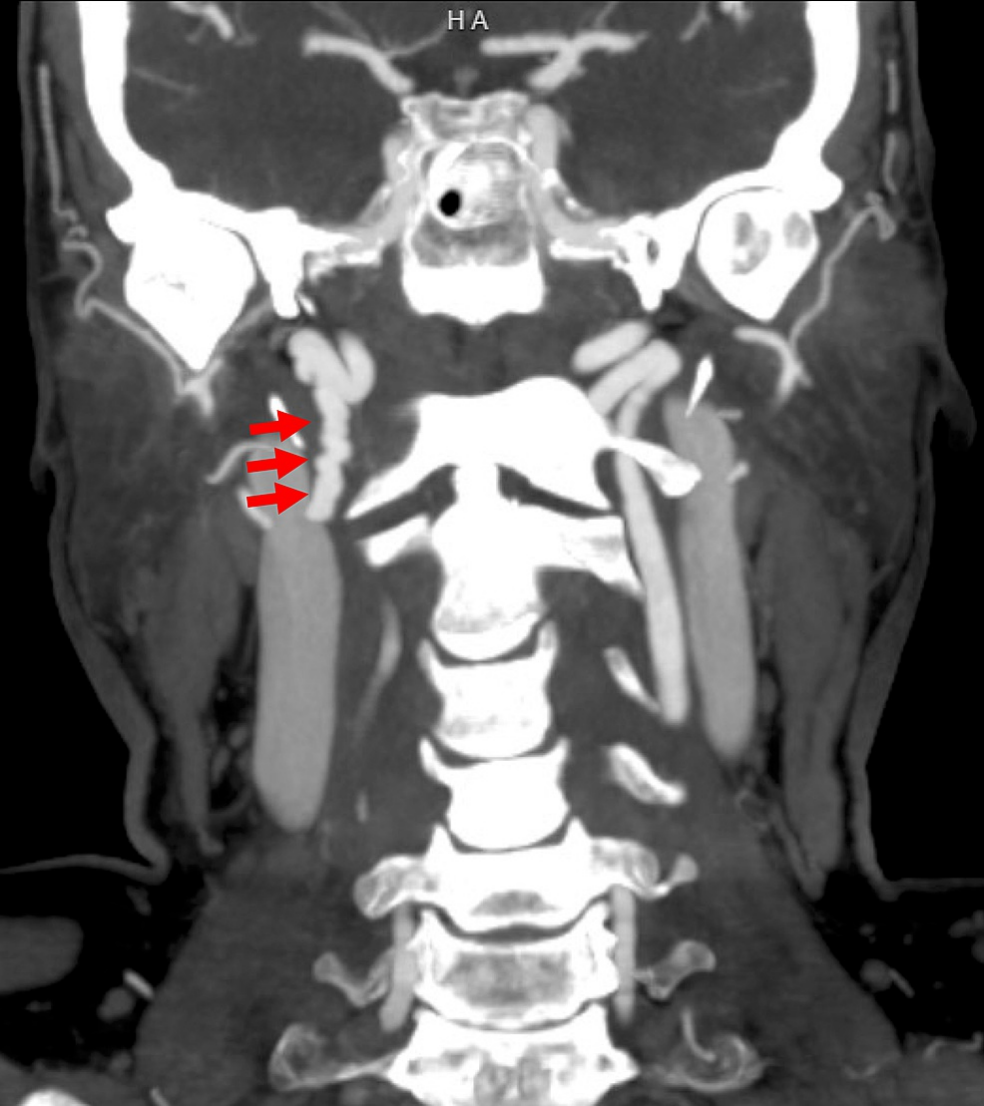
Neck CT angiogram shows coronal maximal intensity projection. Note the irregular beaded narrowing of the mid to distal cervical right ICA consistent with fibromuscular dysplasia.
